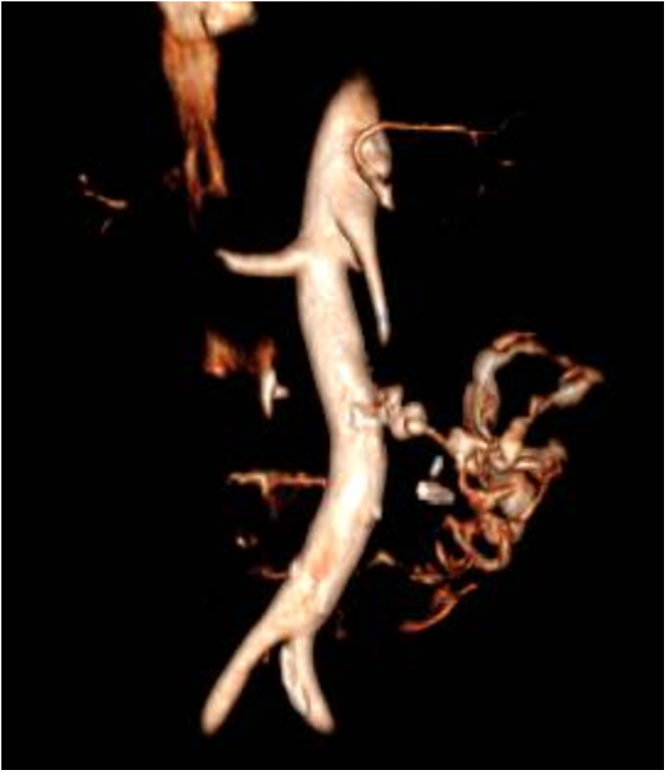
CT scan showing a retroperitoneal perforation near the distal duodenum with an adjacent small abscess near the superior mesenteric artery (SMA).
